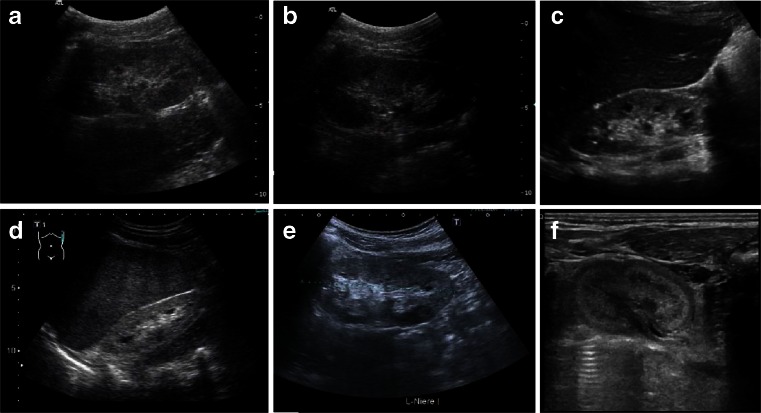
a–f Sonographic appearance of patients of different ages with nephronophthisis (NPHP). All patients show a typical NPHP pattern with normal or small-sized kidneys, enhanced echogenicity of the renal cortex, and reduced cortico-medullary differentiation (progressive with renal failure); cysts (e.g., patient in c) are usually a late sign and occur secondarily after patients have progressed to end-stage renal disease and are typically located at the cortico-medullary junction. a, b 10-year-old boy with homozygous *NPHP1* deletion, serum creatinine of 3 mg/dl, glomerular filtration rate of 20 ml/min and mild arterial hypertension. c, d 10- and 15-year-old patients with progressed clinical course. e 14-year-old boy with Senior–Loken syndrome carrying a homozygous deletion of the *NPHP1* gene. Peritoneal dialysis was started recently. f 2-day-old male patient with sonographic evidence of NPHP, polyuria, and systemic hypertension
